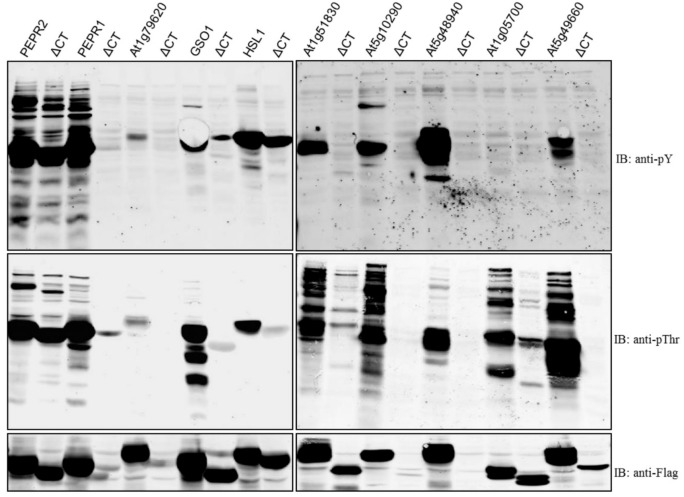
Comparison of wild-type receptor and C-terminal region-deletion kinases (ΔCT) with recombinant proteins of *A. thaliana*. Ten *Arabidopsis* LRR-RLKs were selected based on the length of the amino acid sequence of their CT regions and cloned into the protein expression vector pFlag-Mac. Immunoblots of recombinant full-length and cytoplasmic domain-deletion proteins were probed with pY or pThr antibodies.
